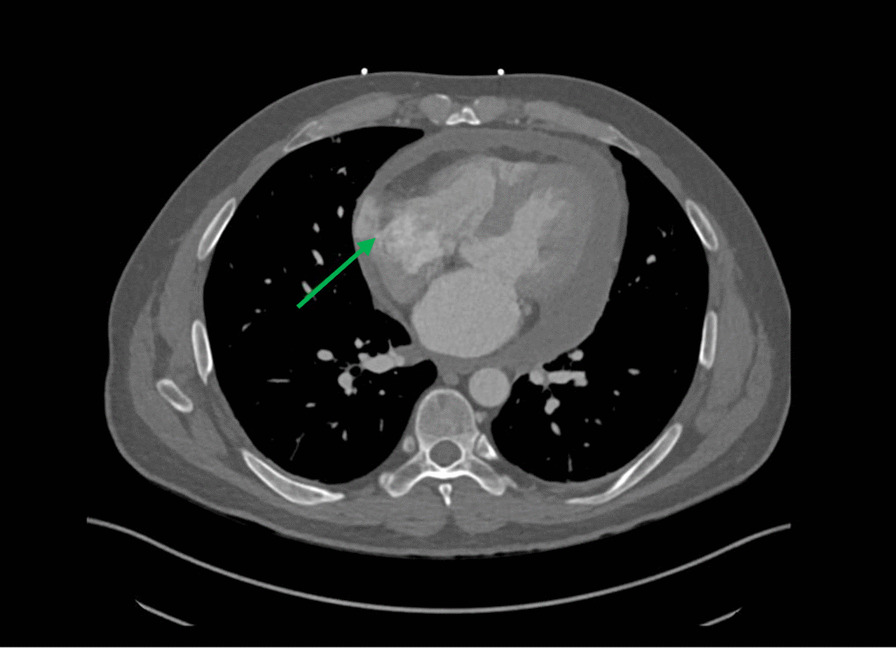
Computed tomography angiogram of the chest. Computed tomography angiogram showing a right atrial pseudoaneurysm. Neck of the pseudoaneurysm is shown by the green arrow. Attenuation of the pericardial fluid was 28.4 Hounsfield units
